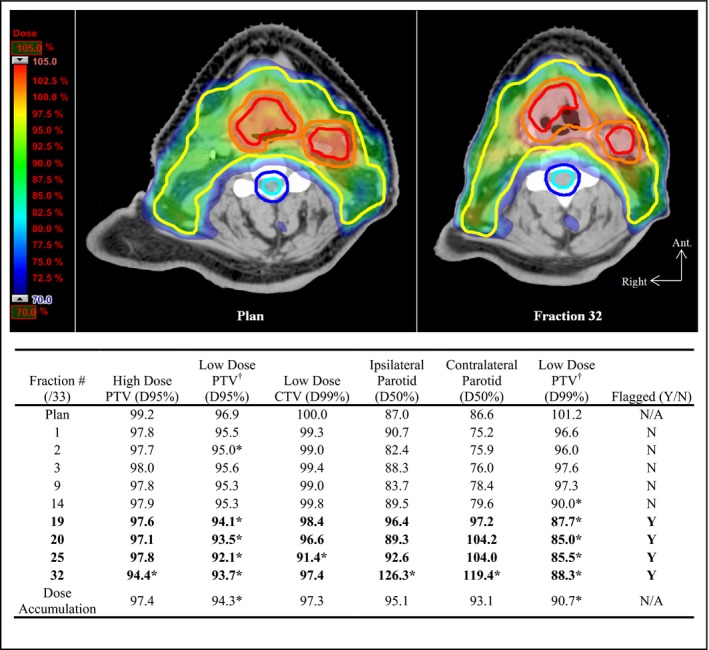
Change in body contour due to general weight loss effects exhibited by Patient 8. Top: pretreatment CT and original plan (left), CT image at fraction 32 and recalculated original plan (right). 70% of the prescription dose (0.70 × 70 Gy = 49 Gy) approximately corresponds to the 3% major violation overdose of the spinal cord (49.4 Gy). Bottom: dose parameters calculated for major and minor violation criteria on fractions with CBCT acquisition. Entries are expressed as a percentage of planning objective or planned parameter value (see Table 2). Bold entries indicate fractions flagged by the protocol. (Contours: red — high‐dose GTV, orange — high‐dose PTV, yellow — low‐dose PTV, cyan — spinal cord, blue — spinal cord with margin). *Clinically significant deviation according to the major/minor violation criteria (only those parameters violating Table 2 criteria are shown). ^†^Low‐dose PTV volume excludes the high‐dose PTV volume.
